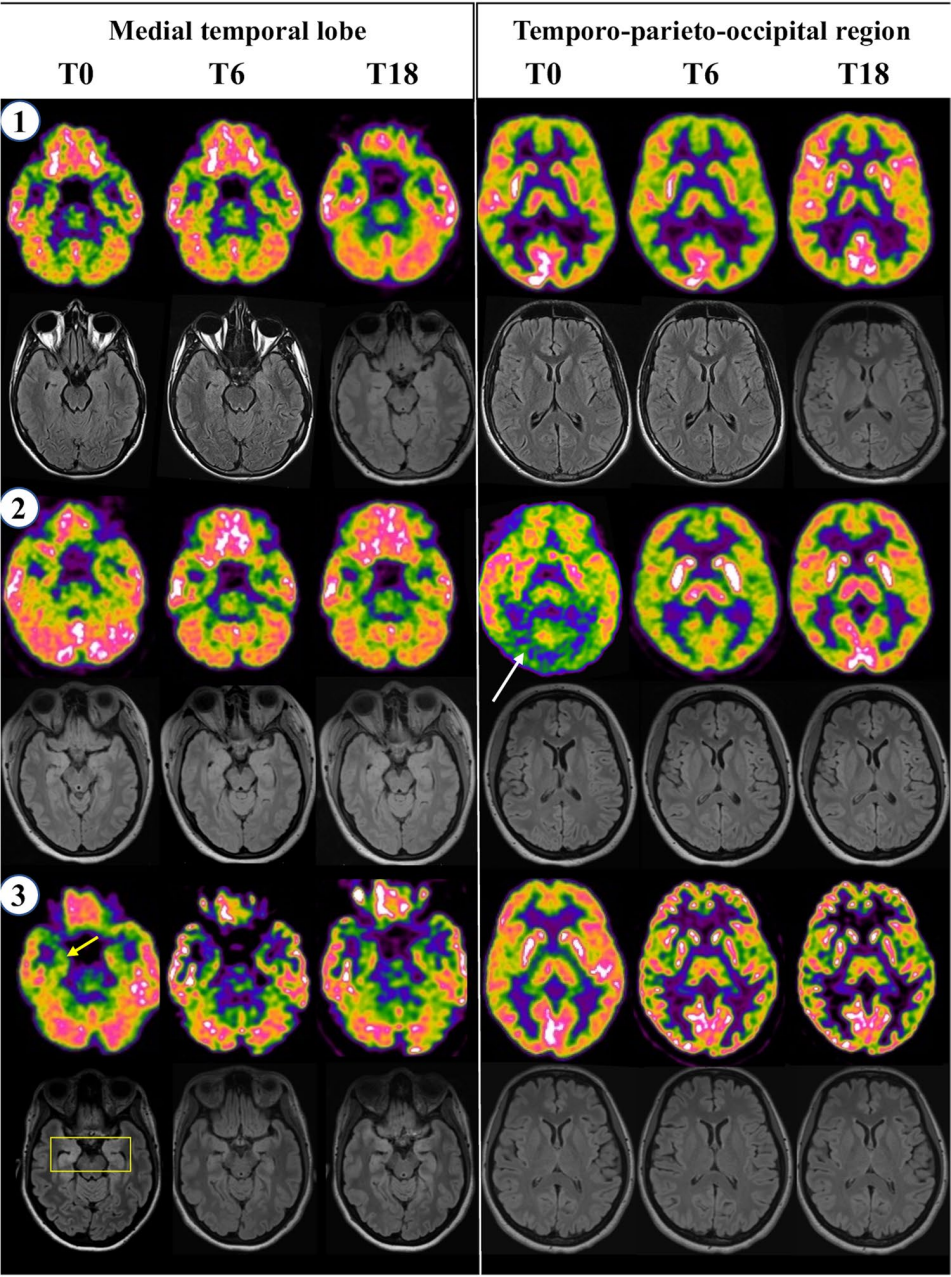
Longitudinal imaging findings in the three patients with NMDAR encephalitis. [^18^F]-FDG-PET (upper parts of the panels) and matching MRI-T2-Flair sequences (lower parts) (patient’s number in the circle). Axial scans display the medial temporal lobe (on the L) and temporo-parieto-occipital region (on the R). MRI showed slight hyperintensity in medial temporal lobes in patient #3 only at T0 (R > L, yellow rectangle), whereas [^18^F]-FDG-PET showed (a) hypometabolism in lateral temporal and posterior parietal lobes bilaterally, and asymmetry (L < R) in frontal-insular and thalamic regions; the abnormalities were more evident at T6, almost entirely solved at T18, with only a slight asymmetry in the frontal-insular cortex and thalamus (patient #1); (b) bilateral occipital hypometabolism at T0 (white arrow), and relative slight hypermetabolism in basal ganglia compared to widespread cortical hypometabolism at T6; only a slight asymmetry (L < R) remained in temporo-polar regions at T18 (patient #2). Metabolism in the medial temporal lobe was unremarkable in both patients #1 and #2 and reduced (R < L) in patient #3 (yellow arrow), matching the MRI-detected hyperintensity; this abnormality was utterly solved at T6-18. L, left; R, right; T, timepoint; numbers, months from onset
